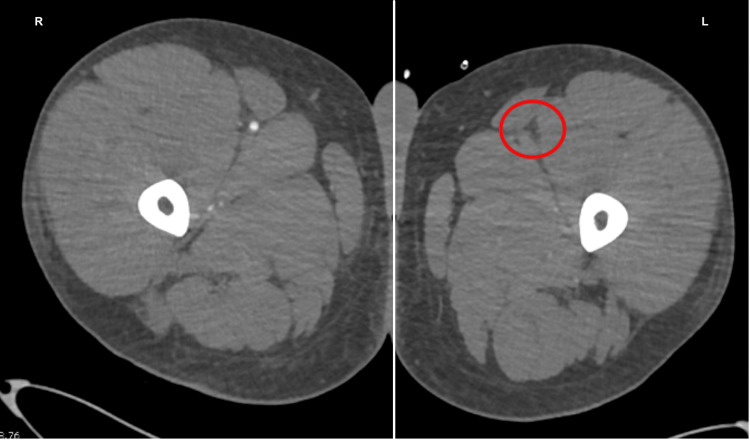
Repeat CT angiogram of both lower limbs showing re-occlusion throughout the left limb CT: computed tomography
